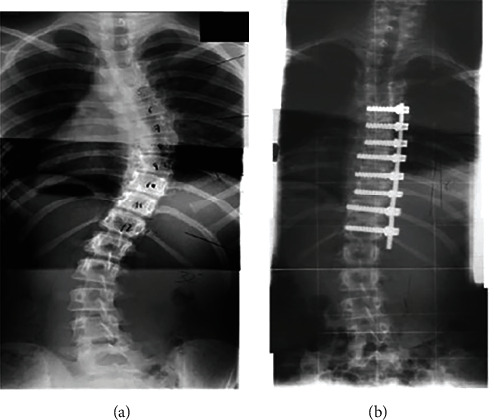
Sample of spine radiographs, (a) preoperatively and (b) postoperatively with having a single rod (anterior procedure) [3].
